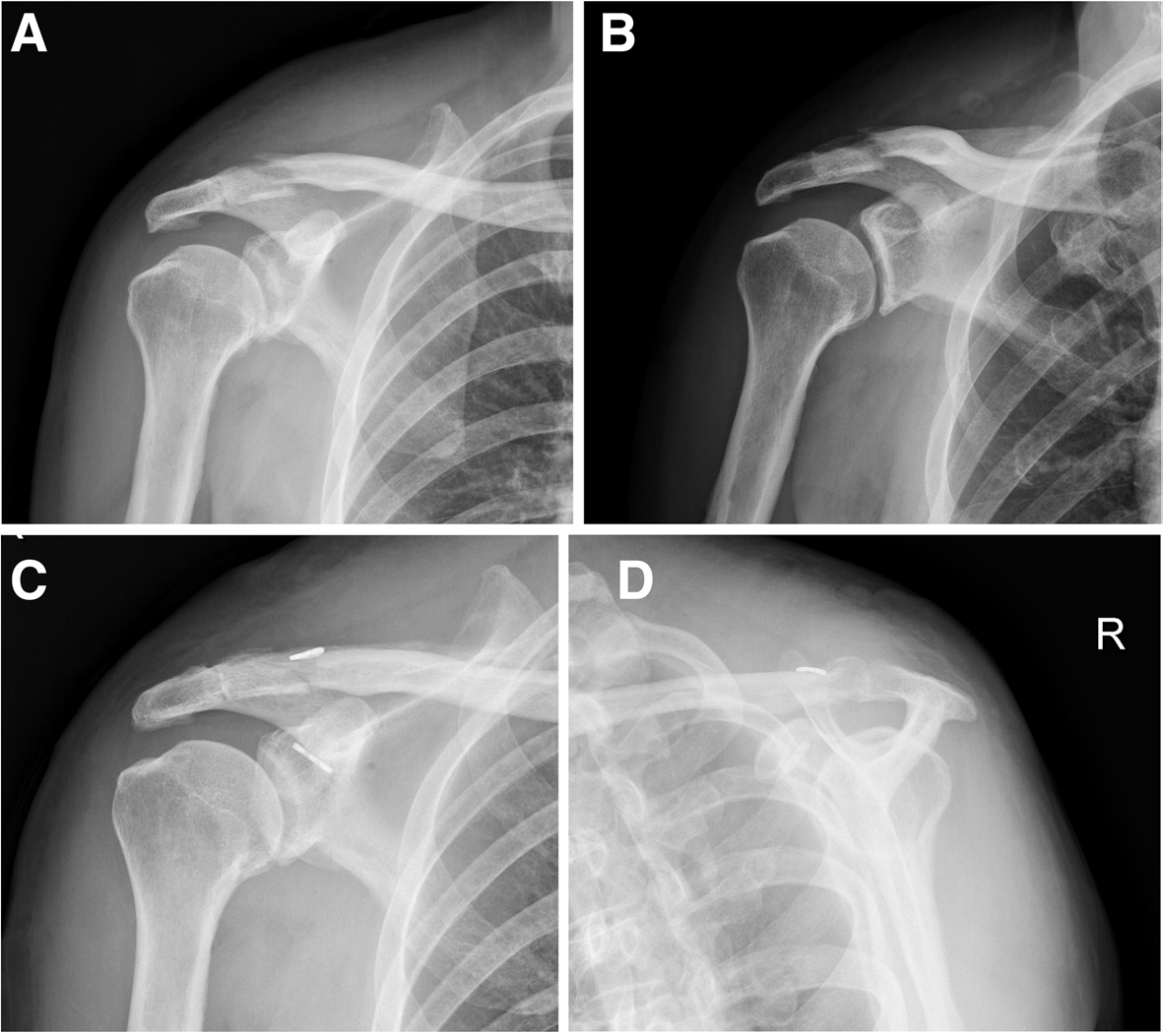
A 31-year-old female with a painful shoulder after falling, (A.B) preoperative radiograph showing Neer IIB distal clavicle fractures, (C.D) postoperative radiograph after treated with arthroscopic assisted fixation using Tight-rope technique
